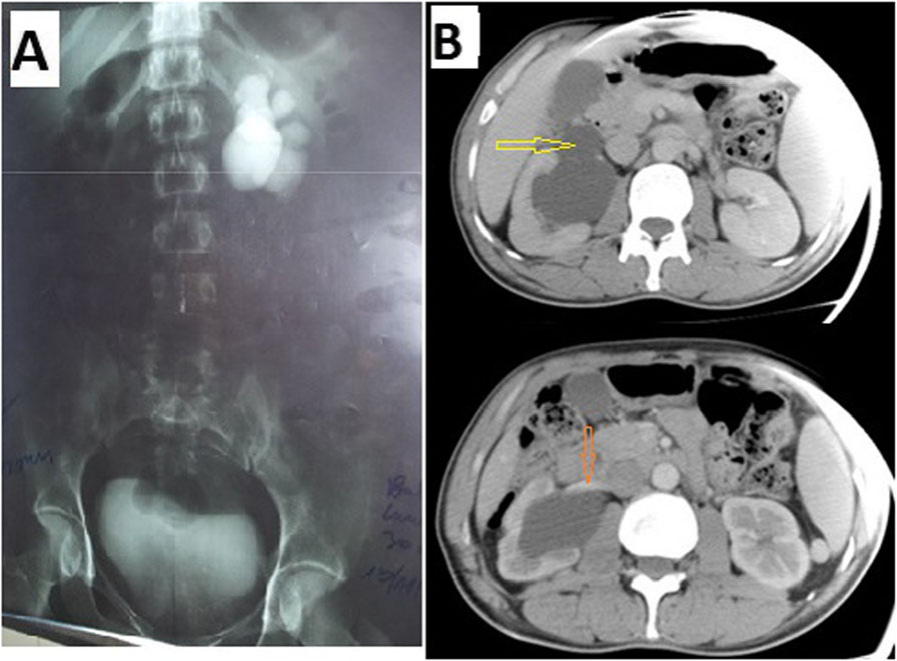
a Intravenous urography: Left pyelic dilation in ball with convex lower edge and non-opacified subpyretic ureter. b Injected abdominal CT scan in axial section showing pyelic dilatation (yellow arrow) compressed in front by a lower polar renal vein (orange arrow)
